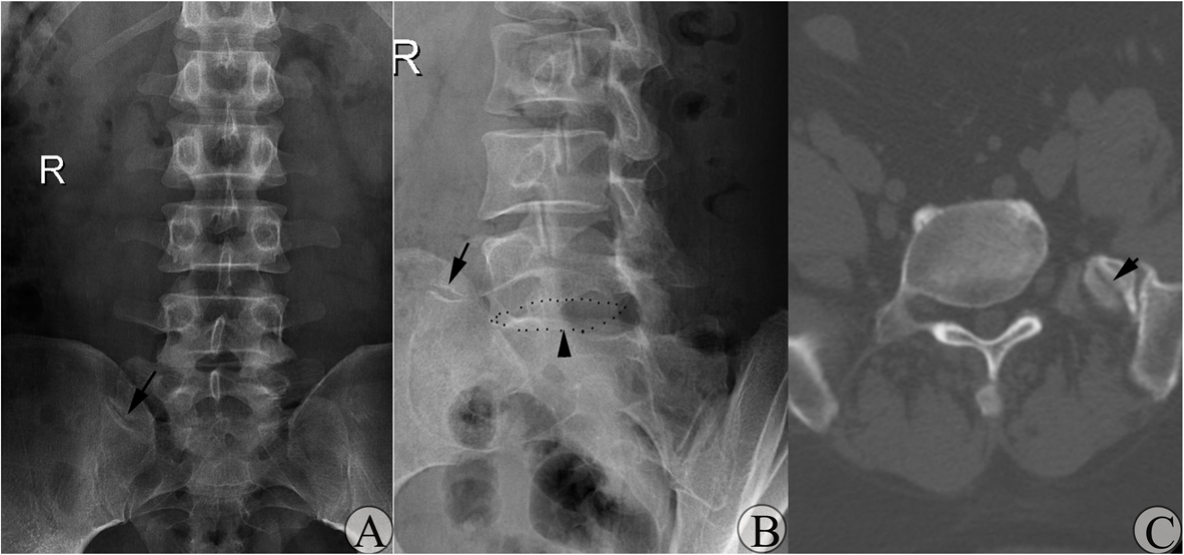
Variance of the orientation of the JLS seen in type IIa MA-LSTV. a AP-LPR image showing that the orientation of the JLS pointed upward when moving laterally instead of parallel to the horizontal line. Arrow indicates the JLS. b Oblique LPR showing orientation of the JBS was not parallel to the inferior endplate of the MA-LSTV. Arrow indicates the JBS. Arrowhead indicates the inferior endplate of the MA-LSTV. c Transaxial CT images showing the orientation of the JBS was not parallel to the coronal plane. Arrow indicates the JLS
